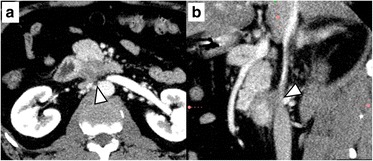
Preoperative computed tomography image of case 1. a Dense soft tissue of the pancreatic tumor contacting the ventral surface of the inferior vena cava (IVC) (*arrowhead*). b Sagittal multi-planar reformation showed that the interface between the tumor and the IVC was not well defined
